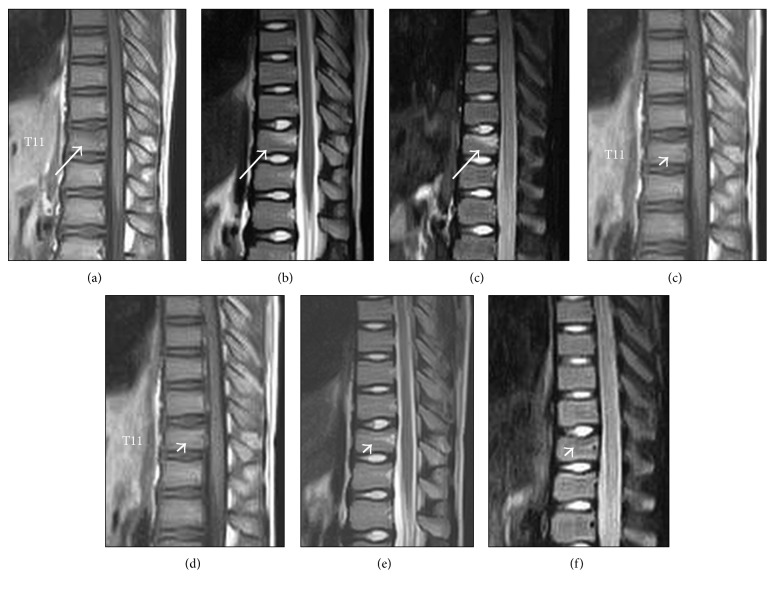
Magnetic resonance images at the initial visit showing low signal on T1WI (a), relatively high signal on T2WI (b), and high signal on short tau inversion recovery (STIR) image (c) in the upper part of the vertebral body at T11 without deformation of the cortical endplate (arrow). At 1-month follow-up later, signal changes had diminished on T1, T2, and STIR images, respectively (d, e, and f, arrowhead).
